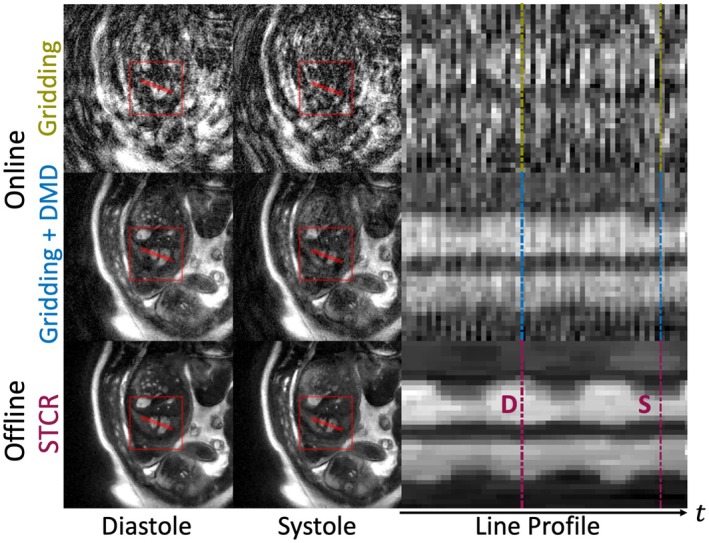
Feasibility of the proposed low‐latency de‐aliasing approach for fetal cardiac RT‐MRI. Shown are end‐diastolic and end‐systolic frames at 21.2 ms (4 TR/frame) temporal resolution. DMD‐based de‐aliasing is applied to the gridded image series; results are shown together with STCR results at the same temporal resolution. Video S7 shows the real‐time movie of the results of the same dataset at 21.2 ms (4 TR/frame) and 42.4 ms (8 TR/frame) temporal resolutions. The proposed reconstruction can perform rapid de‐aliasing to localize the fetal heart with adequate temporal resolution to guide localization.
